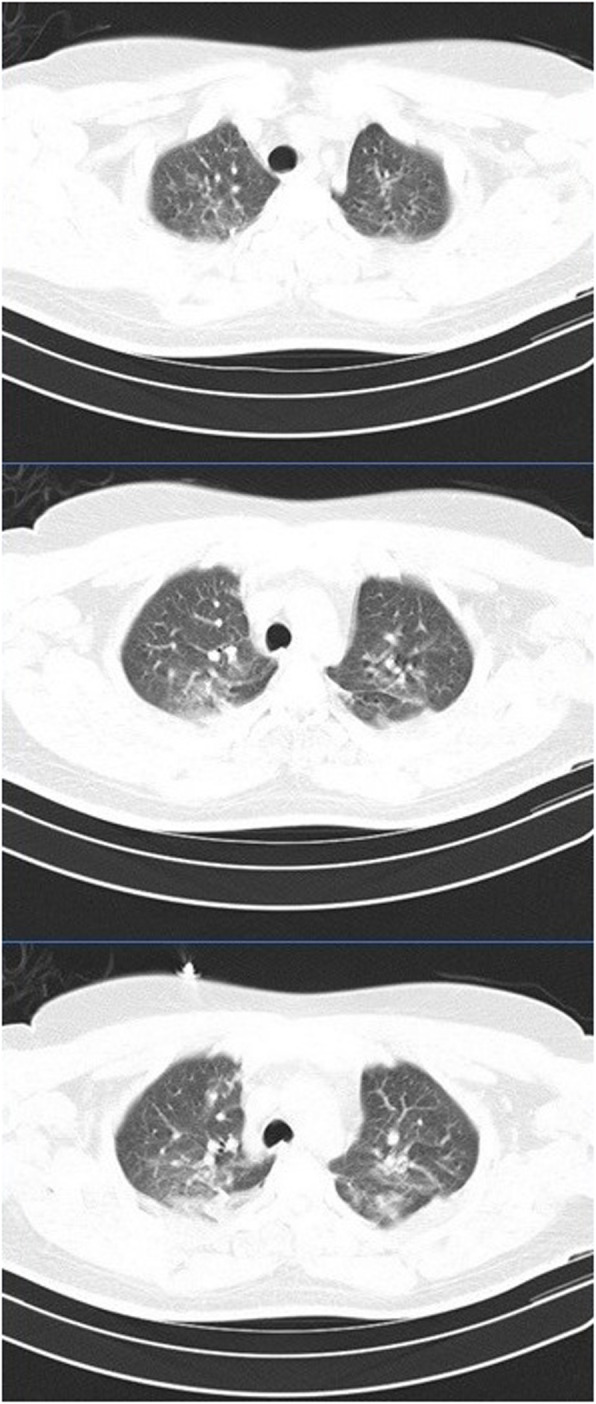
Chest CT did not reveal any lesions such as acute parenchymal injury or pulmonary embolism
